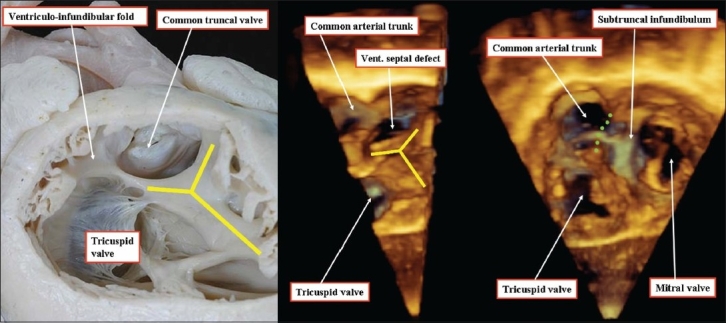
The anatomic image (left hand panel) and the three-dimensional echocardiograms (right hand and middle panels) show how the typical defect seen in the setting of the common arterial trunk is also directly juxta-arterial. In the images shown, there is again a muscular bar interposing postero-caudally between the leaflets of the truncal and tricuspid valves. The defect itself opens to the right ventricle between the limbs of the septomarginal trabeculation or septal band (yellow Y)
